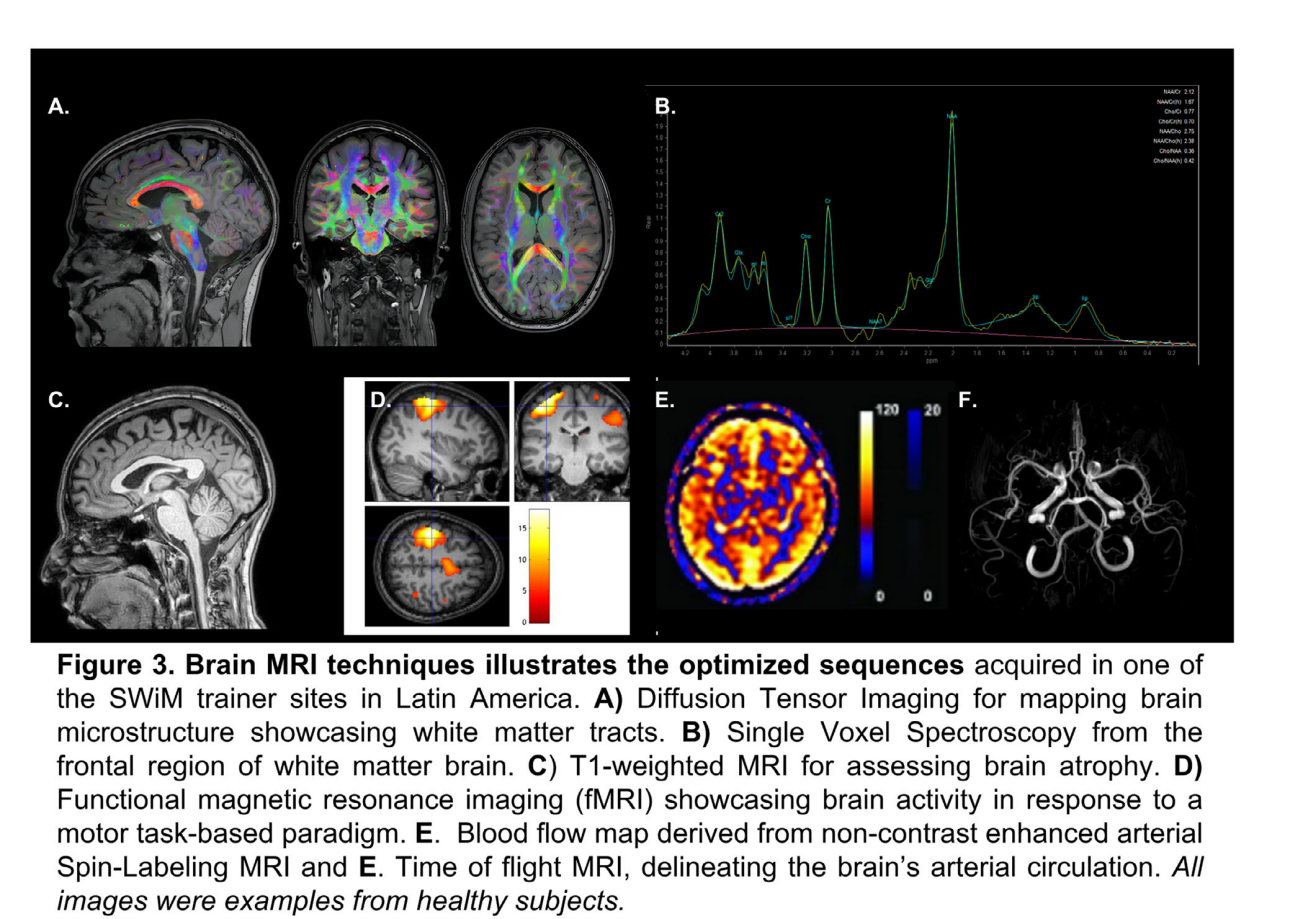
# Enhancing Dementia Imaging in Low‐and‐Middle Income Countries Through Training of Skilled MRI Personnel
